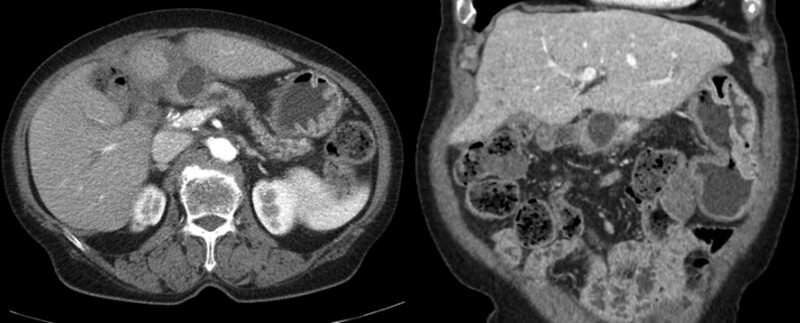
Abdominal computed tomography showed a pancreatic pseudocyst of 3 cm in size and dilated pancreatic duct of the remnant pancreas.
